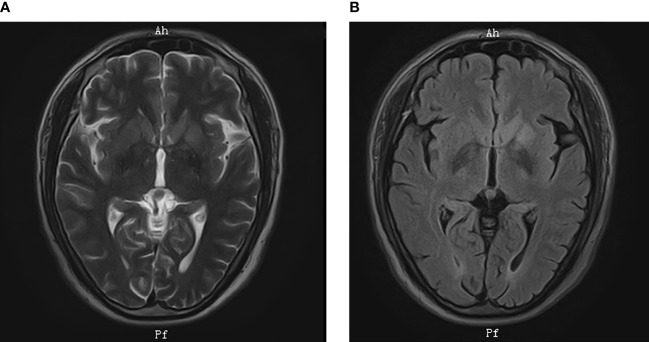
Brain MRI. MRI showed high signal intensity in the left basal ganglia on (A) T2-weighted images; (B) FLAIR sequences.
